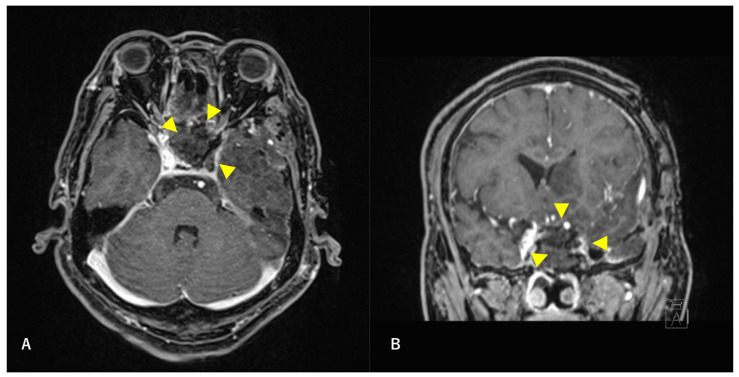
Postoperative T1-weighted contrast-enhanced MRI findings. Axial section (A) and coronal (B) images are shown. The tumor is confirmed to be resected along with the ICA. Arrowheads indicate the site of tumor removal.
